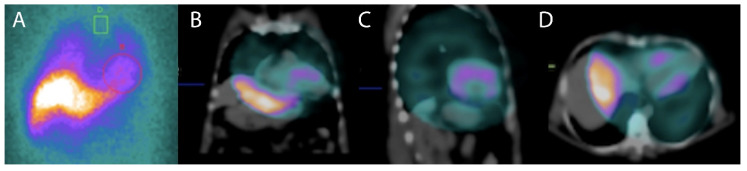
A 123I-MIBG scintigraphy planar scintigraphy in anterior view at 3 h (A) to evaluate the sympathetic innervation a patient with Chagasic cardiomyopathy and ventricular arrhythmia. Heart to mediastinum ratio at 3 h was reduced and radiotracer washout was increased. Single photon emission computed tomography (SPECT)-computed tomography (CT) images in coronal (B), sagittal (C) and transversal (D) planes demonstrated reduced cardiac uptake of 123I-MIBG in apical, inferior and lateral regions indicating sympathetic denervation in these areas and a worse prognosis.
